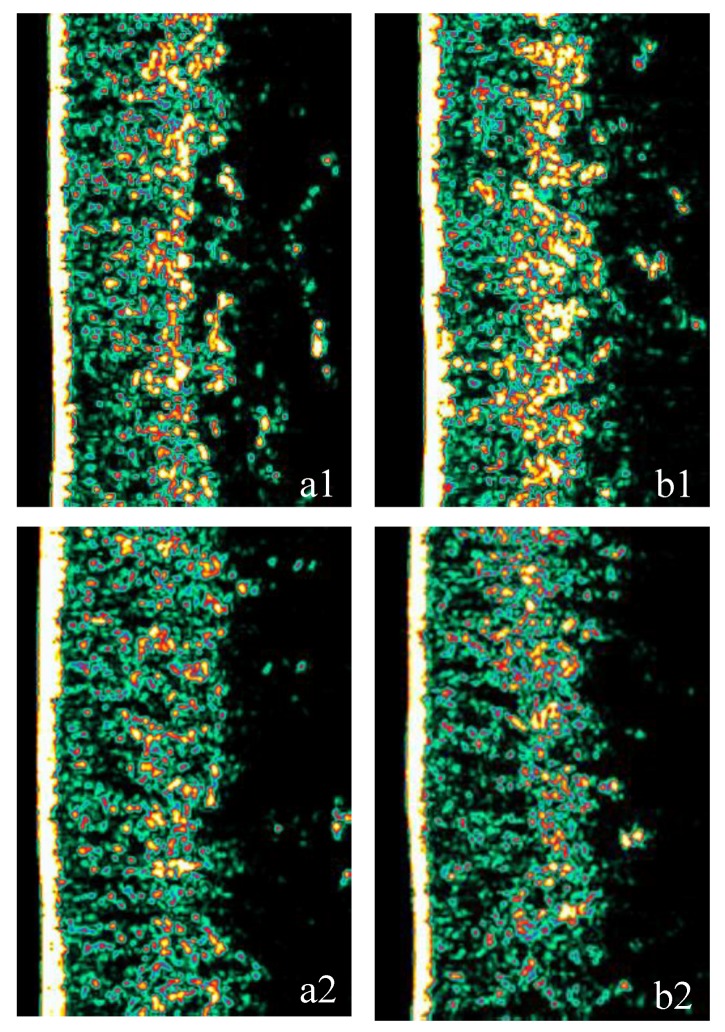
Examples of ultrasound images of the skin before and after 12 weeks of supplementation for a subject from the test group (a1 and b1) and from the placebo group (a2 and b2). In the ultrasound image, the white line to the left represents the epidermis with a water film, to the right followed by dermis, characterized by varying intensities, and subcutis by low-intensity areas due to a homogenous composition. From images a1 and b1, an increase in dermis echogenicity is visible, indicating an improvement in dermal density throughout the study, while slight deterioration can be observed from images (a2) and (b2). Echogenicity colour scale: white > yellow > red > green > black.
